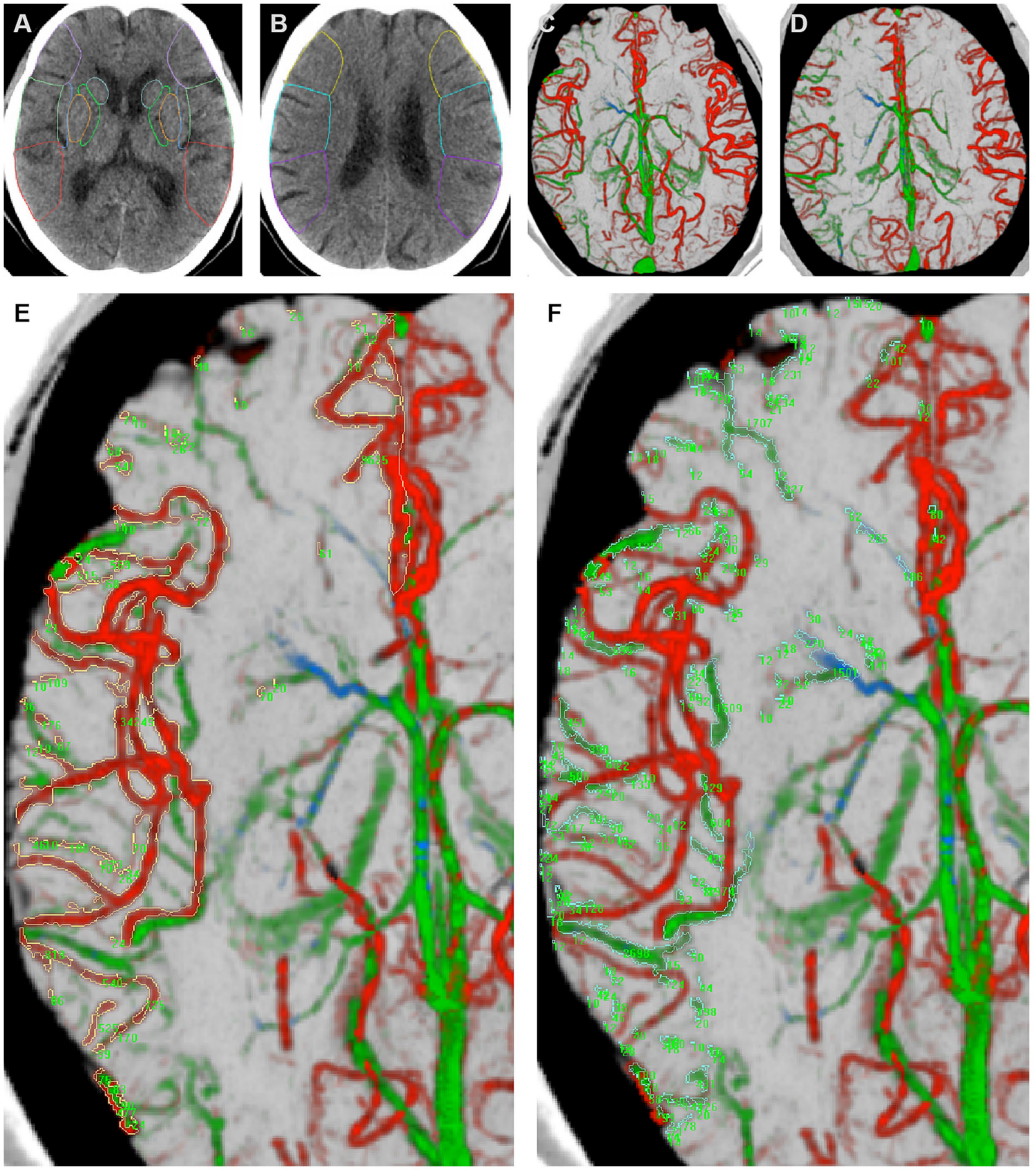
Based on the Alberta Stroke Program Early CT Score regions (A,B) and anterior cerebral artery regions of ColorViz map (C,D), the anterior-circulation “red” vessels (significantly enhanced in the peak artery phase) and “delayed” vessels (“green” and “blue” vessels, significantly enhanced in the peak venous phase and late venous phase) on the affected side of patients with acute ischemic stroke were delineated (E,F).
